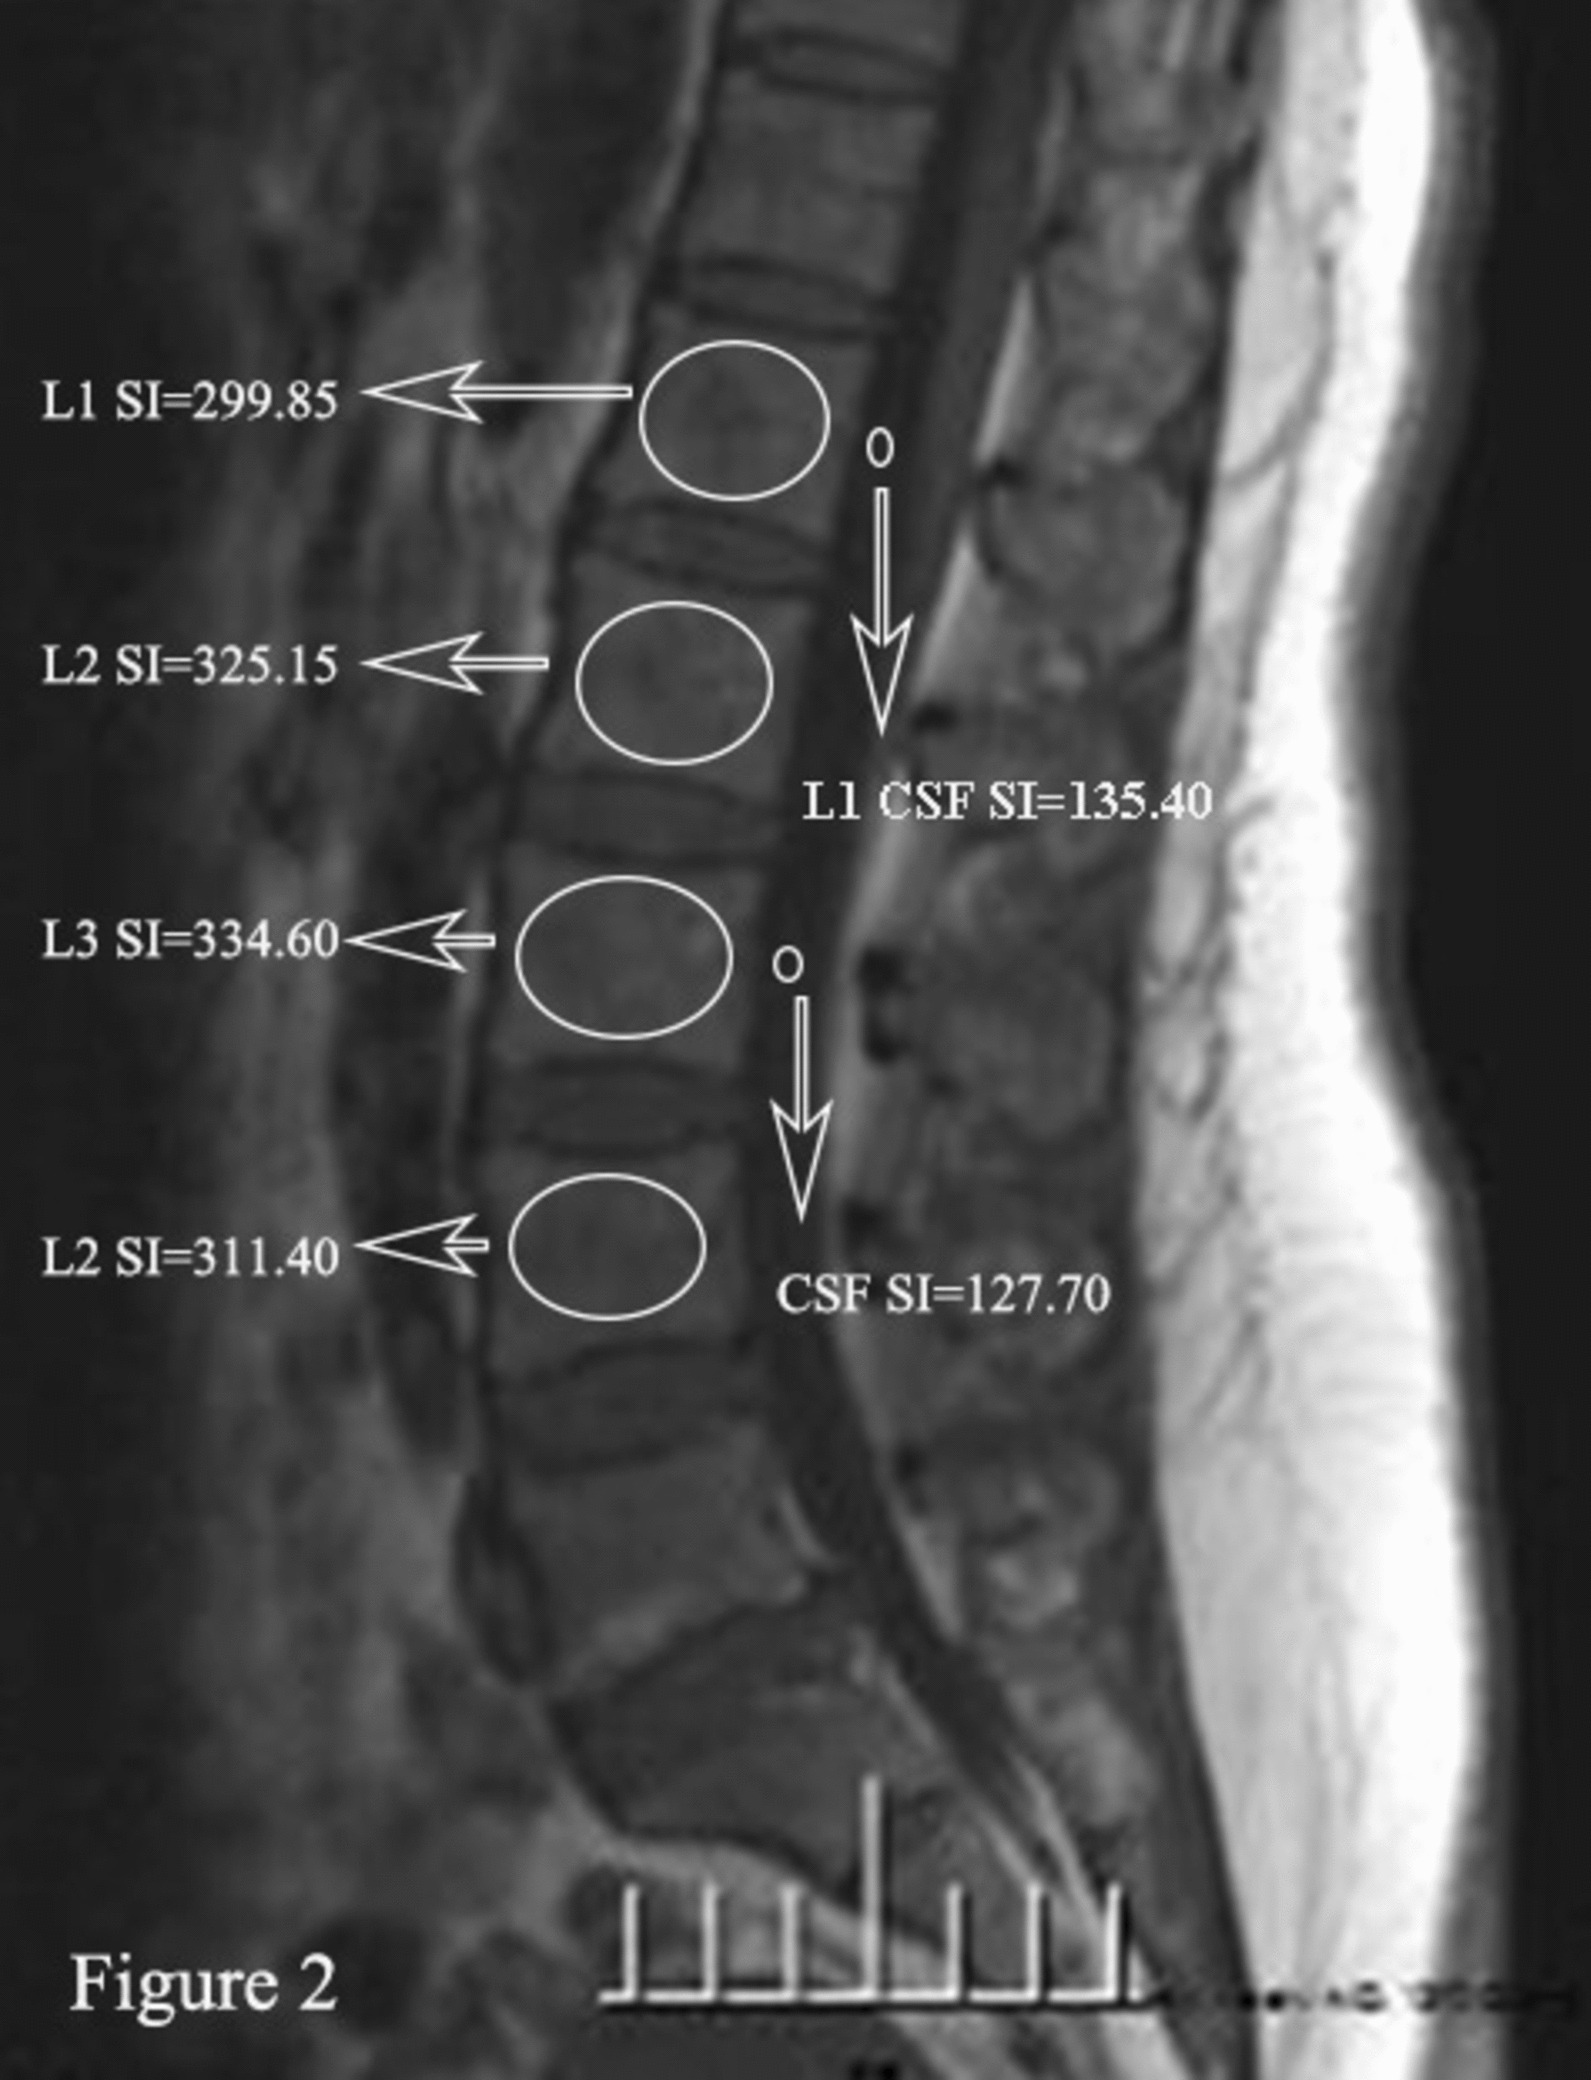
Non − contrast-enhanced T1-weighted MRI image shows the determination of signal intensity (SI) of L1-L4 and SI of cerebrospinal fluid (CSF) at the L1 level and L3 level using regions of interest (ROIs)
